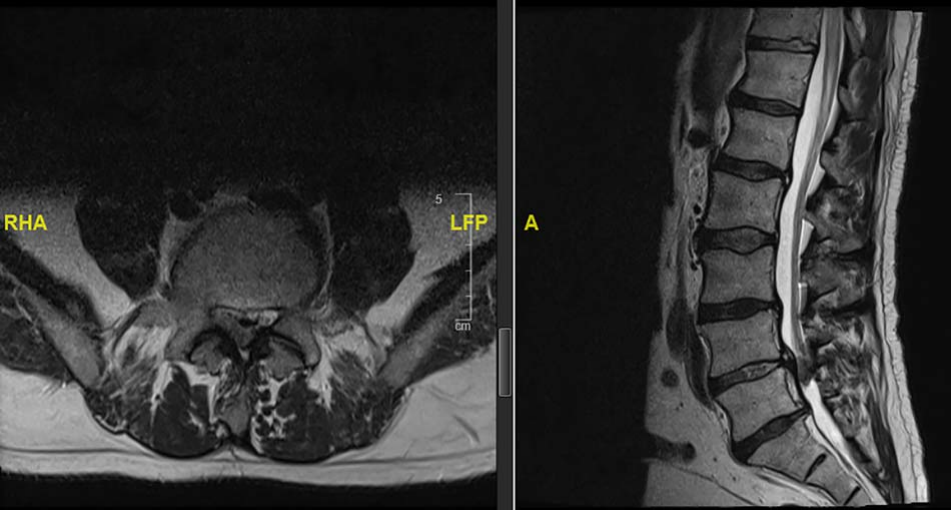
MRI scan showing an intradural disc at L4/5.
